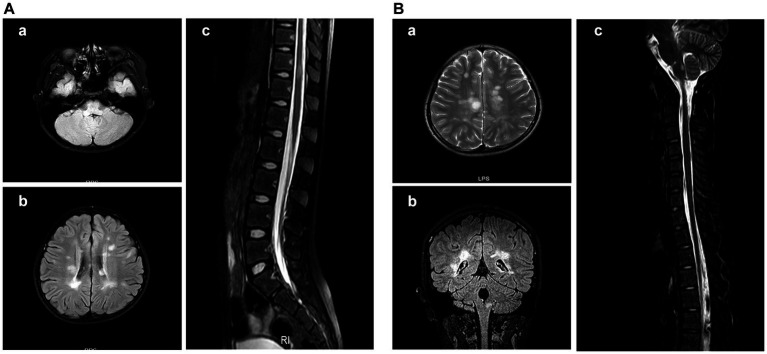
Cranial MRI results of the patient with area postrema syndrome. A female child, aged 133 months, presented with bouts of vomiting and dizziness for over 10 days. (A) Cranial MRI scan at admission. (A-a) Abnormal signal in the right side of the medulla oblongata in the FLAIR sequence. (A-b) Abnormal signal in the bilateral periventricular white matter in the FLAIR sequence. (A-c) Patchy abnormal signal at the T11 level in the FLAIR sequence. (B) Cranial MRI at 30 months after the first MRI scan. The patient complained about headache and had vomited for 2 days. (B-a) The periventricular semioval center of the lateral ventricle in the FLAIR sequence. (B-b) The right medulla oblongata and periventricular white matter in the coronal section of the FLAIR sequence. (B-c) Abnormal signal in the dorsal side of the medulla oblongata in the FLAIR sequence.
